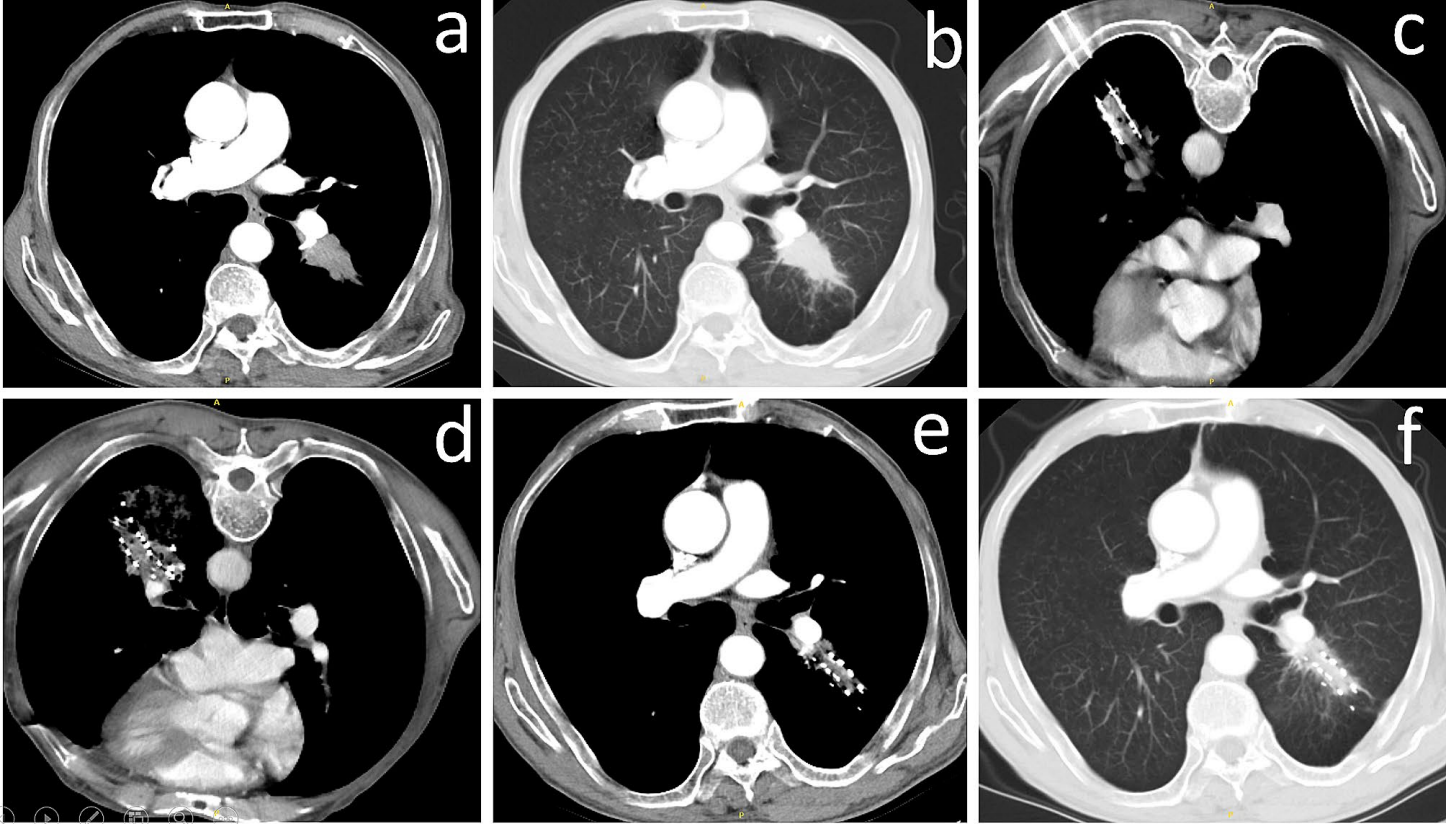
(a) Thoracic CT showed a lesion adjacent to pulmonary artery in the left lung; (b) Lung window;(c) Multiple needles were inserted into the lesion under CT guidance;(d) Iodine-125 seeds were implanted into the tumor;(e) Local control was observed during a Follow-up of 34 months;(f) Lung window
